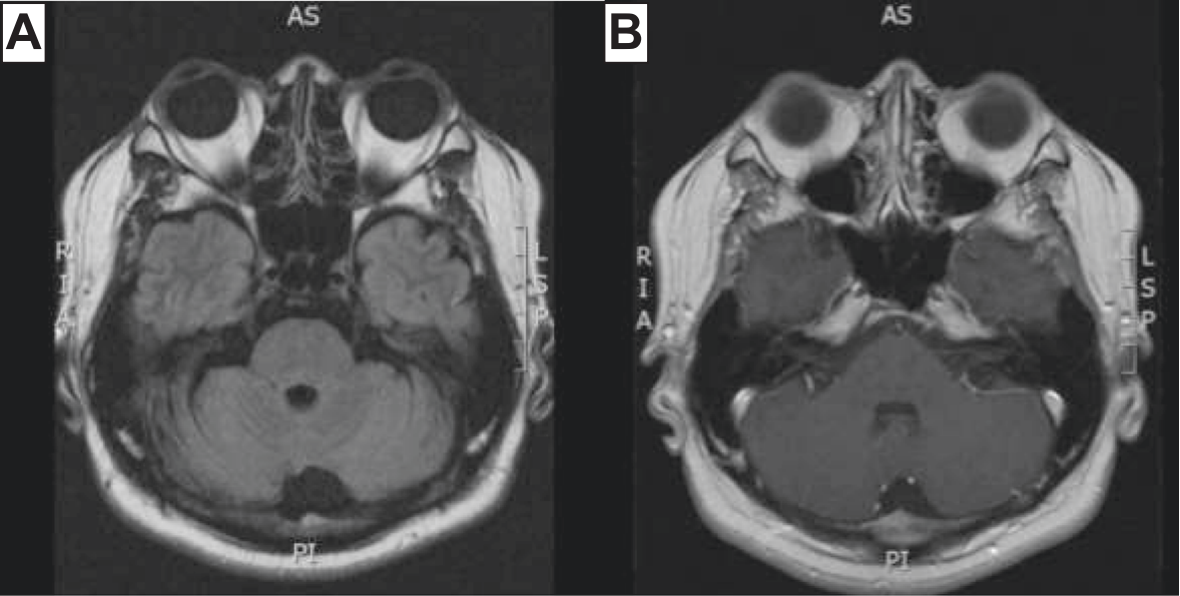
Images obtained 6 months after corticosteroid treatment. a Fluid-attenuated inversion recovery image. b Postcontrast T1-weighted image showing nearly complete resolution of the patient’s pontine lesions
